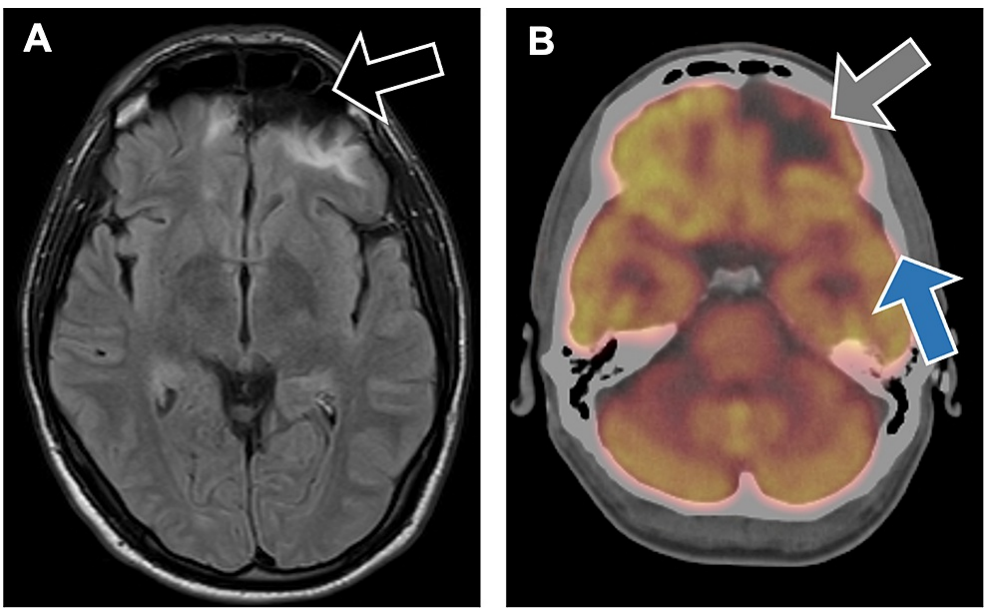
A. MRI brain scan revealing encephalomalacia of both inferior frontal lobes (black arrow) and left anterior temporal lobes related to the patient's traumatic brain injury. B. Positron emission tomography brain scan demonstrating photopenia in the left frontal lobe (gray arrow) and to a lesser extent in the left temporal (blue arrow) and right medial frontal lobe.
